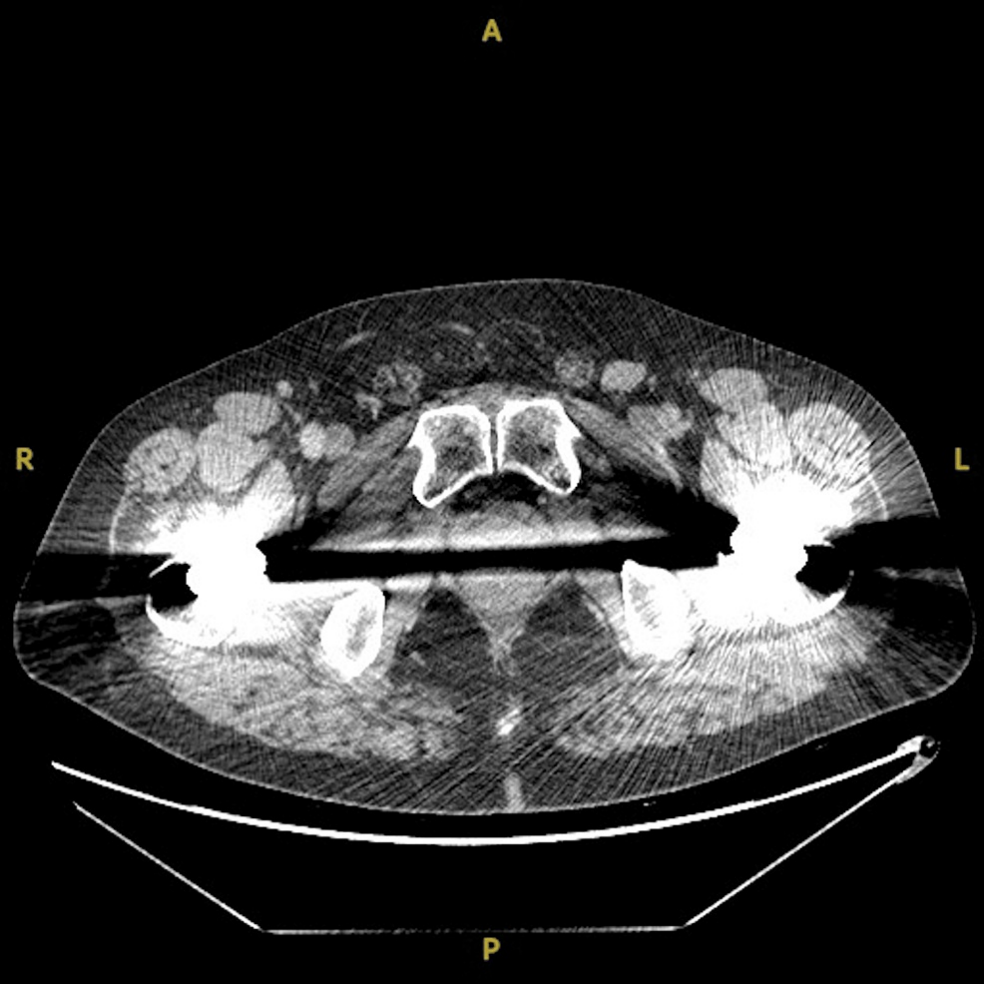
Pre-salvage radiotherapy CT chest/abdomen/pelvis demonstrating inguinal lymphadenopathy. CT: computed tomography
